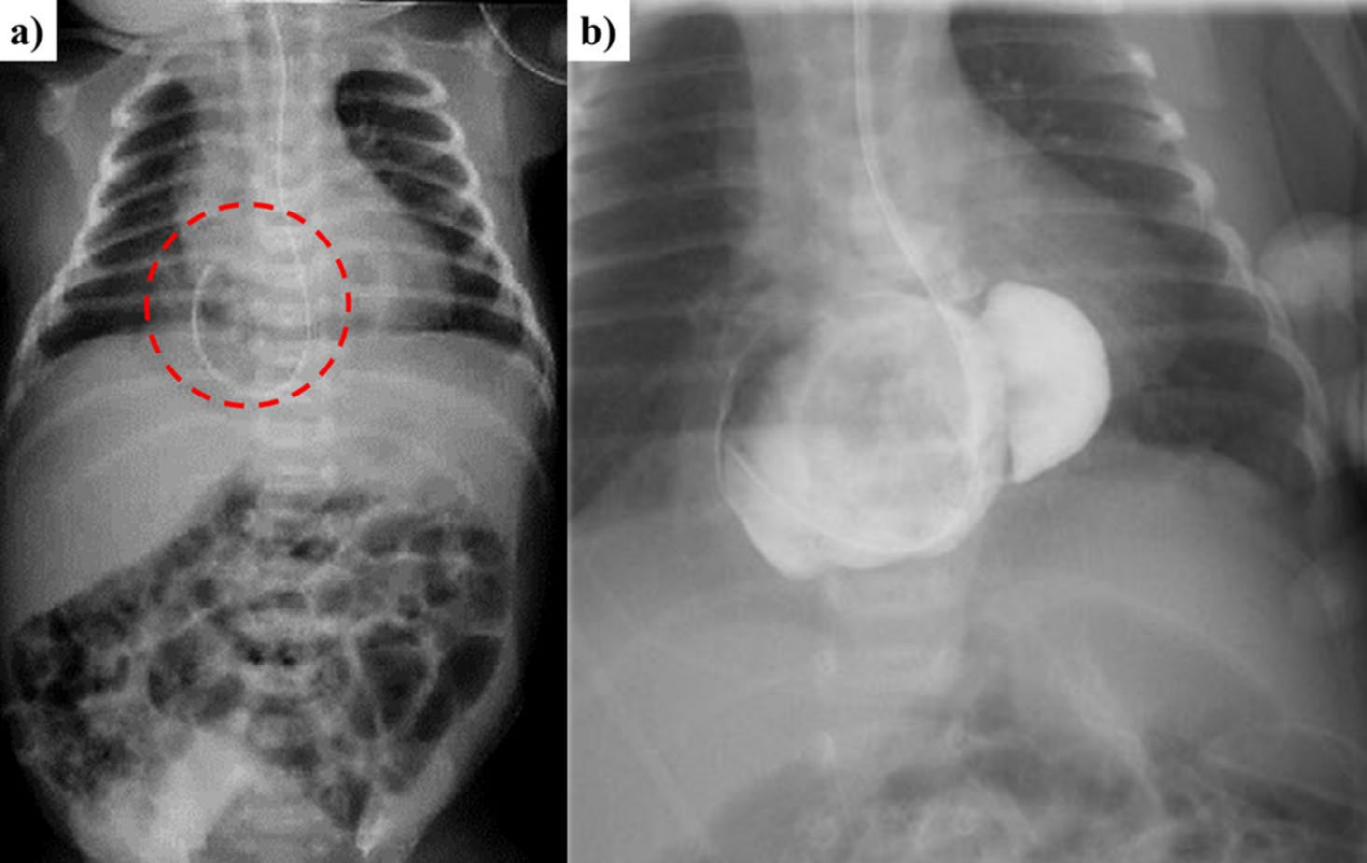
Preoperative radiography findings. (a) Plain radiography shows a gastric tube coiled up at the diaphragm level (red circle). (b) Contrast radiography showed a hiatal hernia with the entire stomach prolapsing into the mediastinum.
